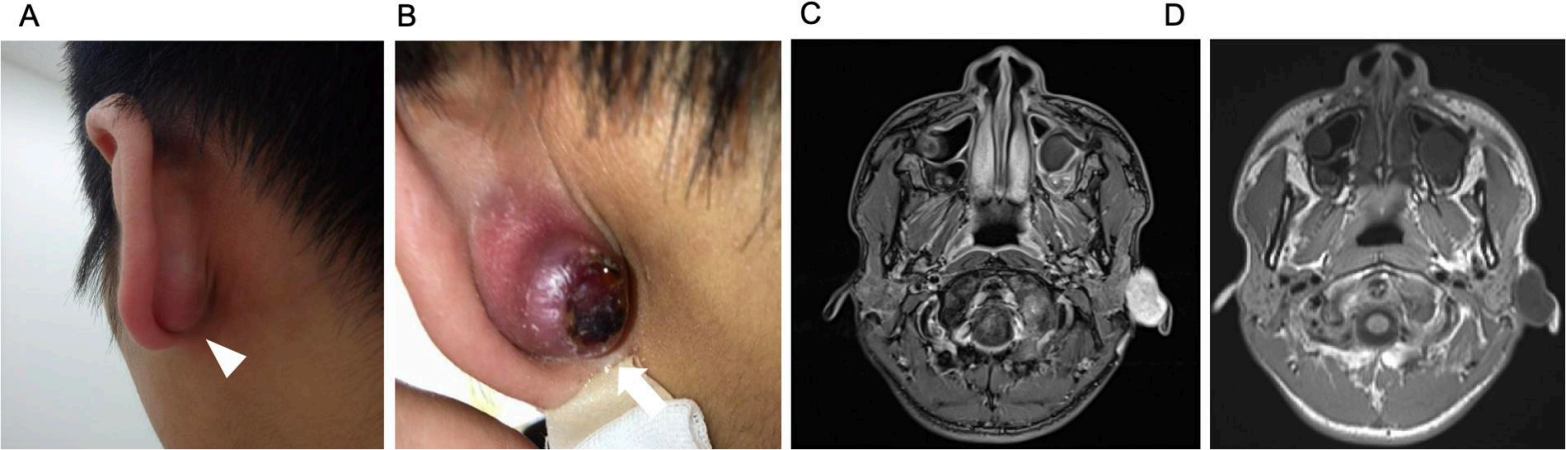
Preoperative clinical and radiologic findings. (A) Clinical photograph of the left postauricular region showing a progressively enlarging, painless mass (arrowhead). (B) Clinical photograph of the lesion, which had gradually increased in size over approximately 6 months and extended anteriorly to involve the preauricular area. Note overlying skin discoloration and central necrotic area (arrow). (C) Shows contrast-enhanced T1-weighted MRI, and (D) shows non-contrast T1-weighted MRI.
